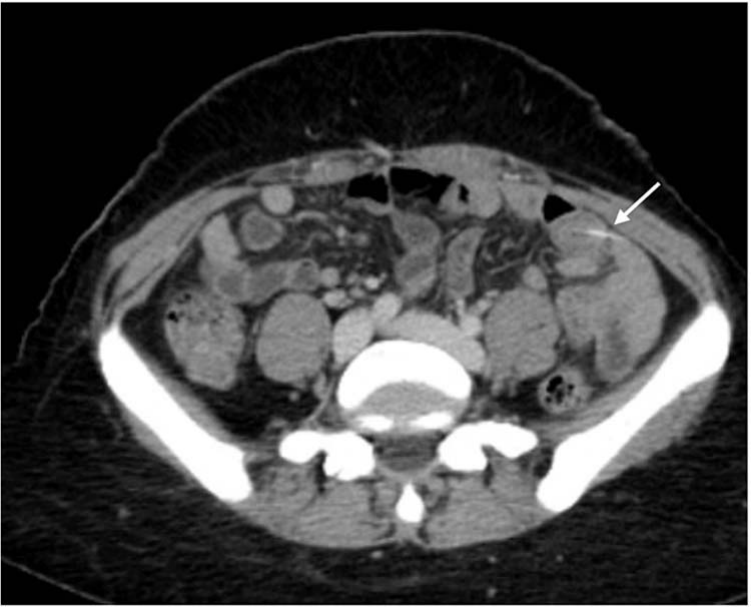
Axial views of contrast-enhanced CT of the abdomen showed a transversely oriented linear hyperdense FB (arrow) structure seen within the ilea loops in the lower quadrant.
